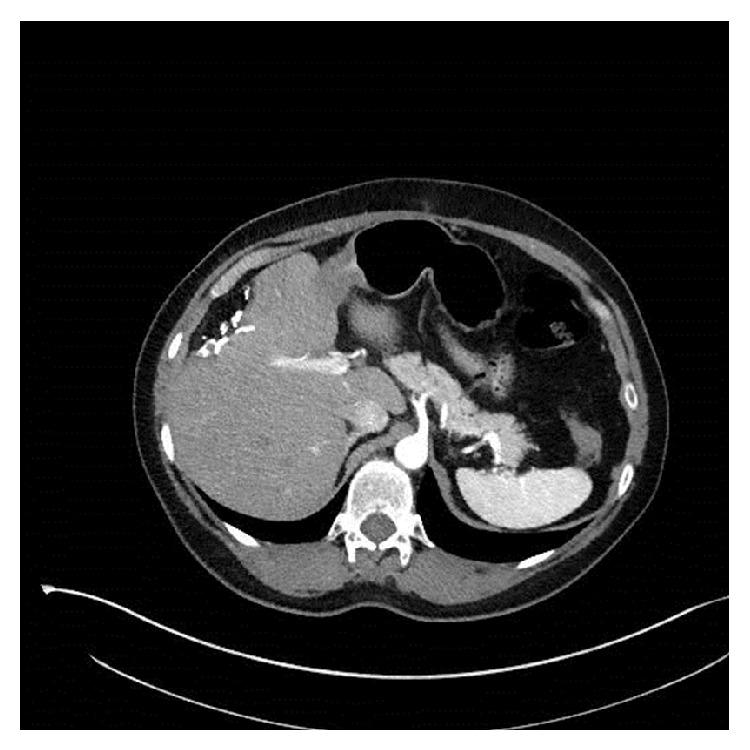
Axial view of follow-up CT scan showing resection margin and no evidence of recurrence.
